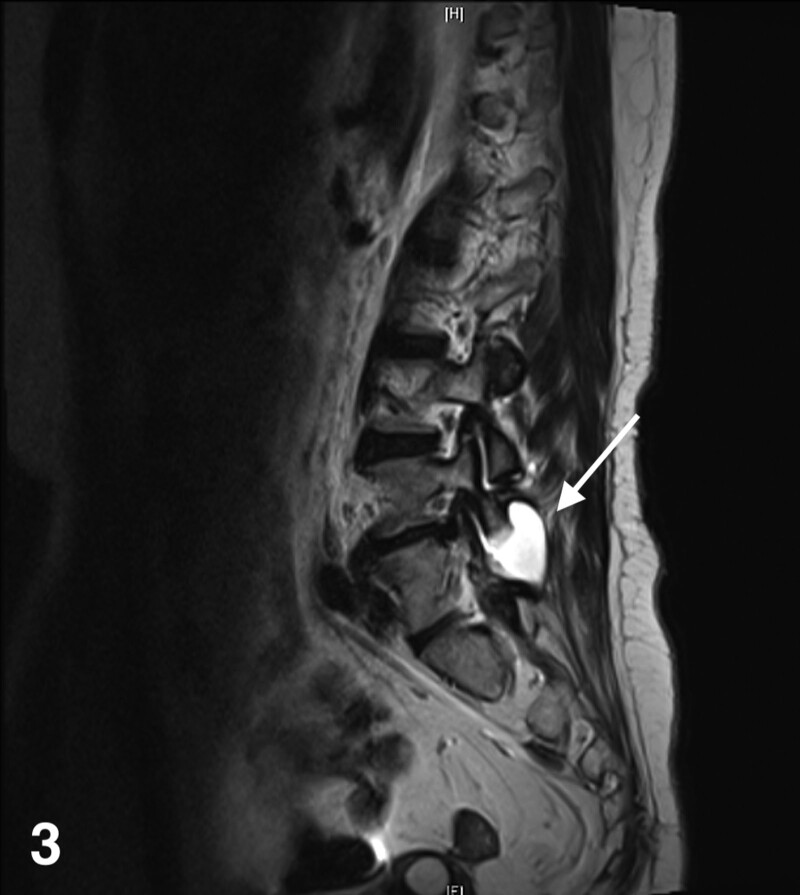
Sagittal MRI of lumbar spine in patients after UBE surgery. MRI = magnetic resonance imaging.
